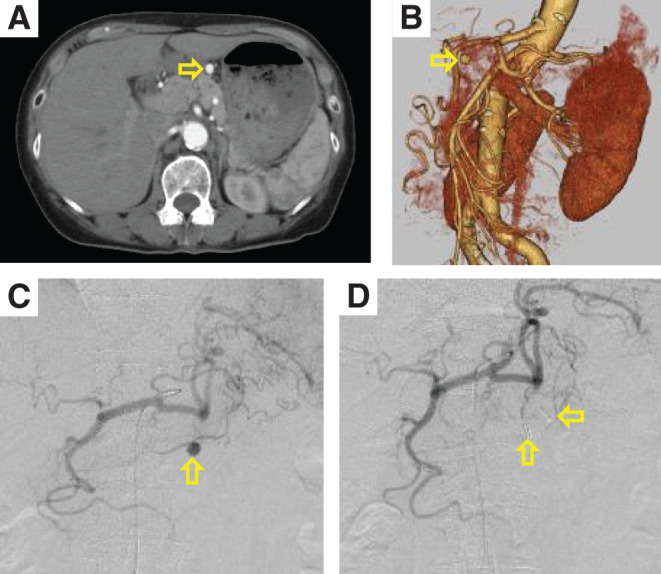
Fig. 1 Preoperative CT and intraoperative angiography. (A) Sagittal view of the right gastric artery measuring 8 mm (arrow). (B) Three-dimensional reconstructed CT image of the right gastric artery (arrow). (C) Angiography of the celiac artery showing the right gastric artery (arrow). (D) The aneurysm in the right gastric artery was completely resected. Arrows indicate the clips on the inflow and outflow arteries. CT: computed tomography
